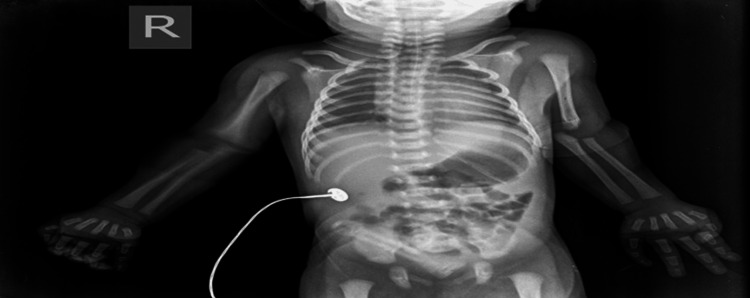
Radiograph of the upper limbs revealing normal morphology of the distal phalanges with no evidence of hypoplasia or aplasia
